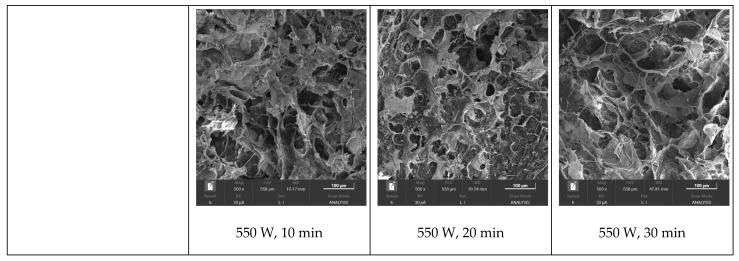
Effect of ultrasound power and treatment time on hardness (A), WHC (B) and morphology by scanning SEM (C) of coffee cherry. Note: ns indicates a non-significant difference between infusion time in a control sample (*p* > 0.05). NS indicates a non-significant difference between infusion time in an infused sample (*p* > 0.05).  indicates a significant difference between control and infused sample at the same infusion time (*p* < 0.05).
